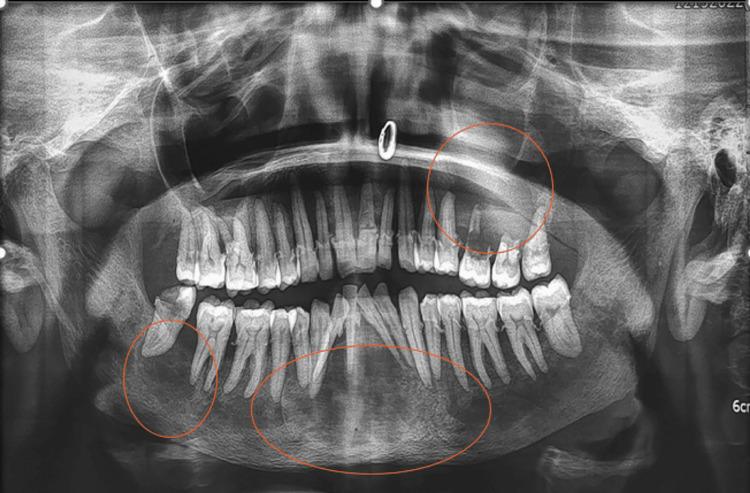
Orthopantomogram Orthopantomogram (OPG) revealing a generalised loss of lamina dura. Well-defined radiolucencies with non-corticated borders are noted in the left posterior maxillary and right posterior mandibular regions. Another well-defined, unilocular radiolucency with corticated borders showing heterogenous radiolucent to radio-opaque features is seen in the mandibular symphysis.
